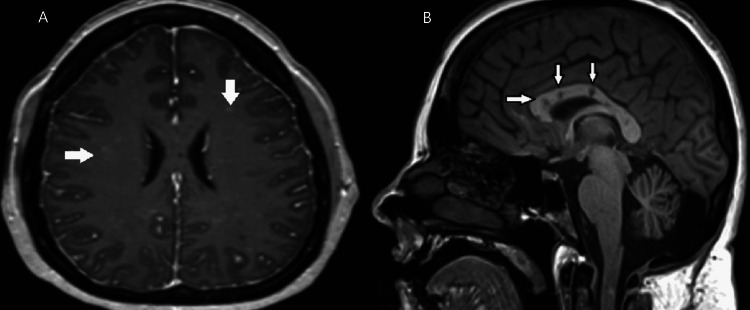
Axial (A) and sagittal (B) T1-weighted magnetic resonance image demonstrating central callosal punched-out lesions (white arrows)
